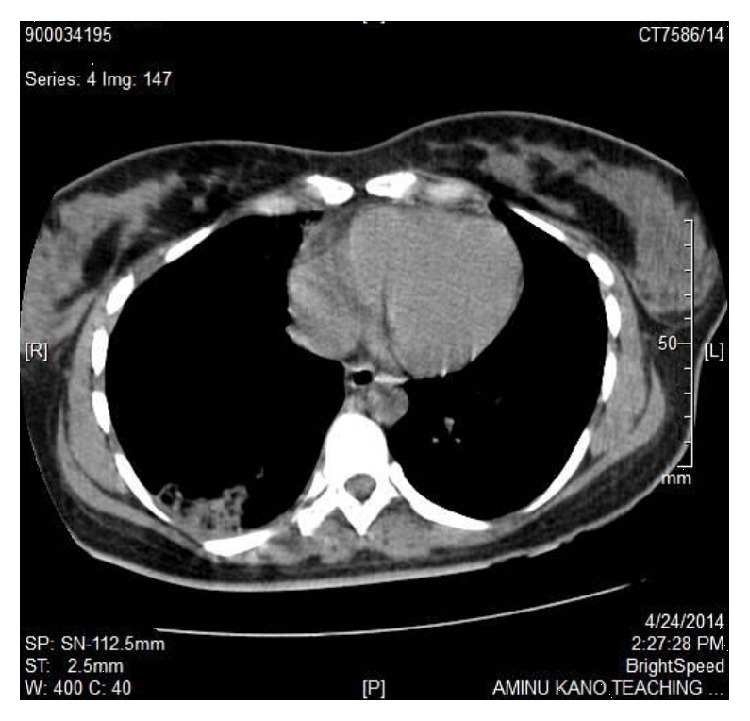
Chest CT scan showing consolidation with “cavities” in the right posterior lung base.
